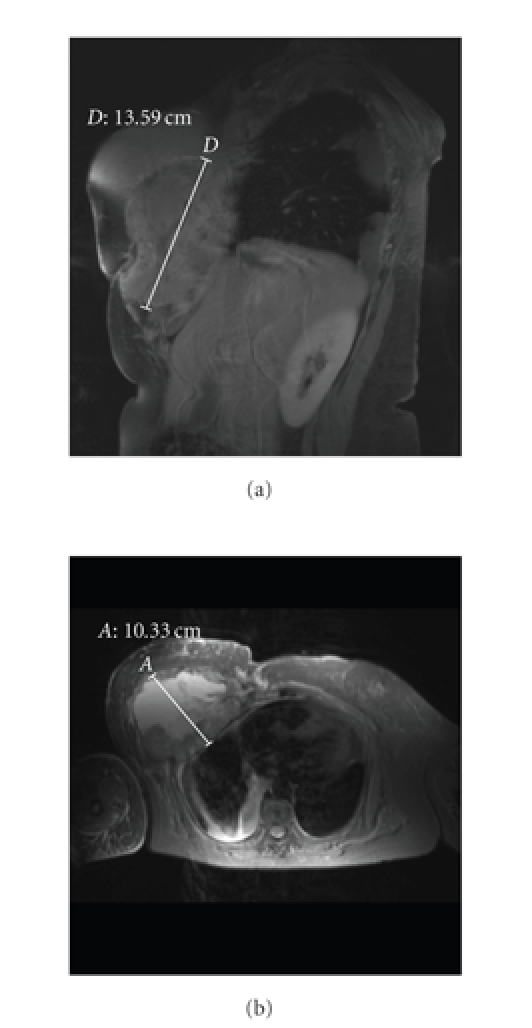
(a) MRI: tumor growth on right breast area. (b) MRI: myocutaneous flap infiltration.
